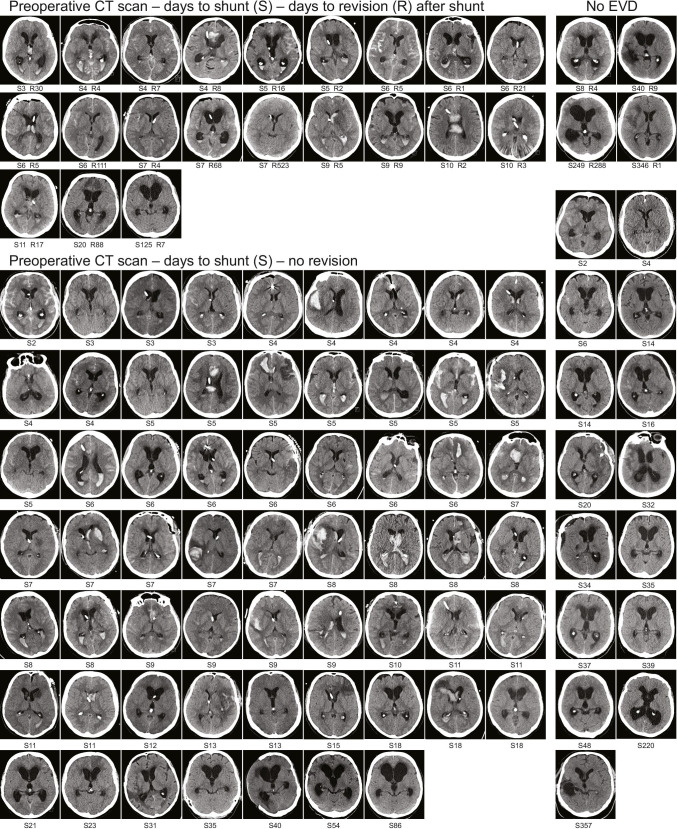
Representative CT slices of 101 aSAH patients before shunt insertion. The 101 consecutive aSAH patients with a ventriculoperitoneal shunt (adjustable valve) inserted from 2010 to 2018 (Table 2 and Fig. 3). The figure shows one representative CT slice from the last available imaging of each patient before the shunt insertion. Under each CT slice are the days to the shunt insertion (S) since aSAH. A total of 25 patients had a shunt revision (three upper rows) with the days to the shunt revision (R) under their CT slices. EVD (extraventricular drainage) was installed in 82 patients (nine left columns). Due to EVD or spinal drainage, some ventricles are small at the time of the shunt insertion, and in some cases, EVD is removed and followed by spinal drainage
